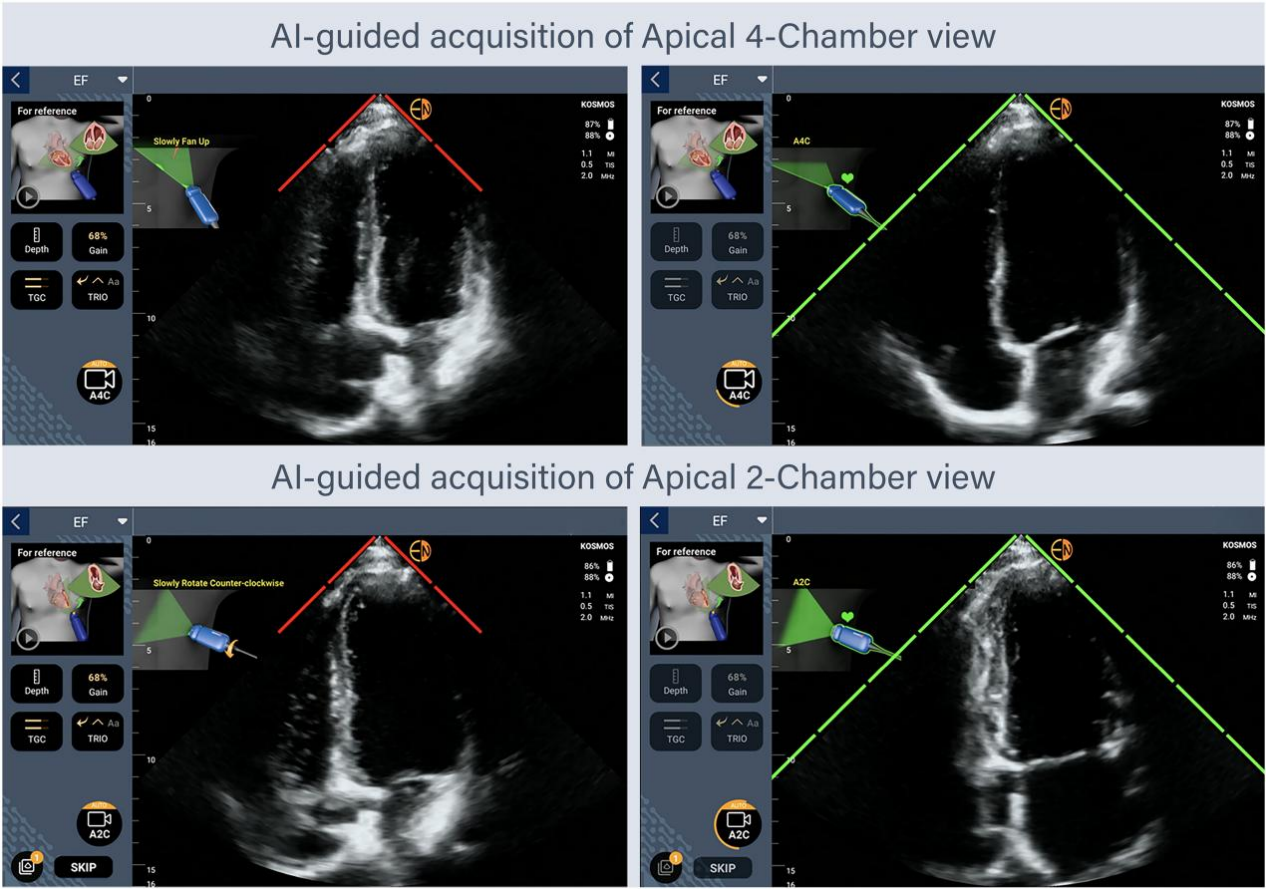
Artificial intelligence-assisted acquisition of optimal apical four-chamber and two-chamber echocardiographic views using the GUIDANCE and GRADING functions of the Kosmos TRIO AI software. A2C, apical two-chamber; A4C, apical four-chamber; AI, artificial intelligence.
